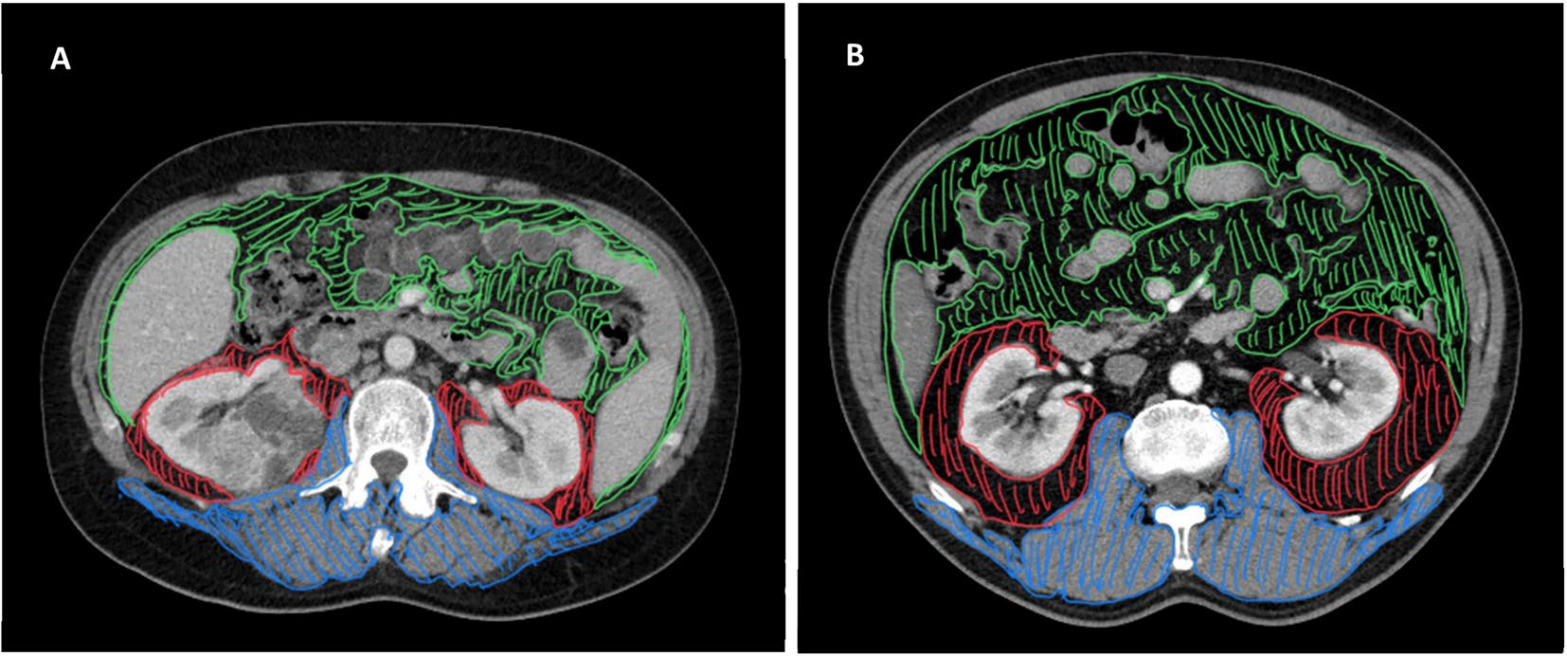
Representative examples of adiposity measures using CT scan. Axial images at the level of renal hilum from a non-obese (A) and an obese patient with RCCa (B). VAT is represented in green, PRAT in red and skeletal muscles in blue. VAT, visceral adipose tissue area; PRAT, perirenal adipose tissue; CT, computed tomography.
